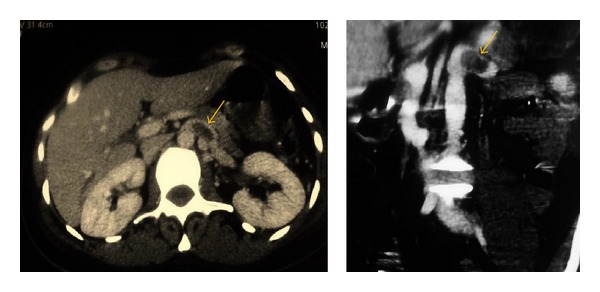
CT of the abdomen and pelvis demonstrating a dilated left renal vein with intraluminal thrombus (yellow arrow).
